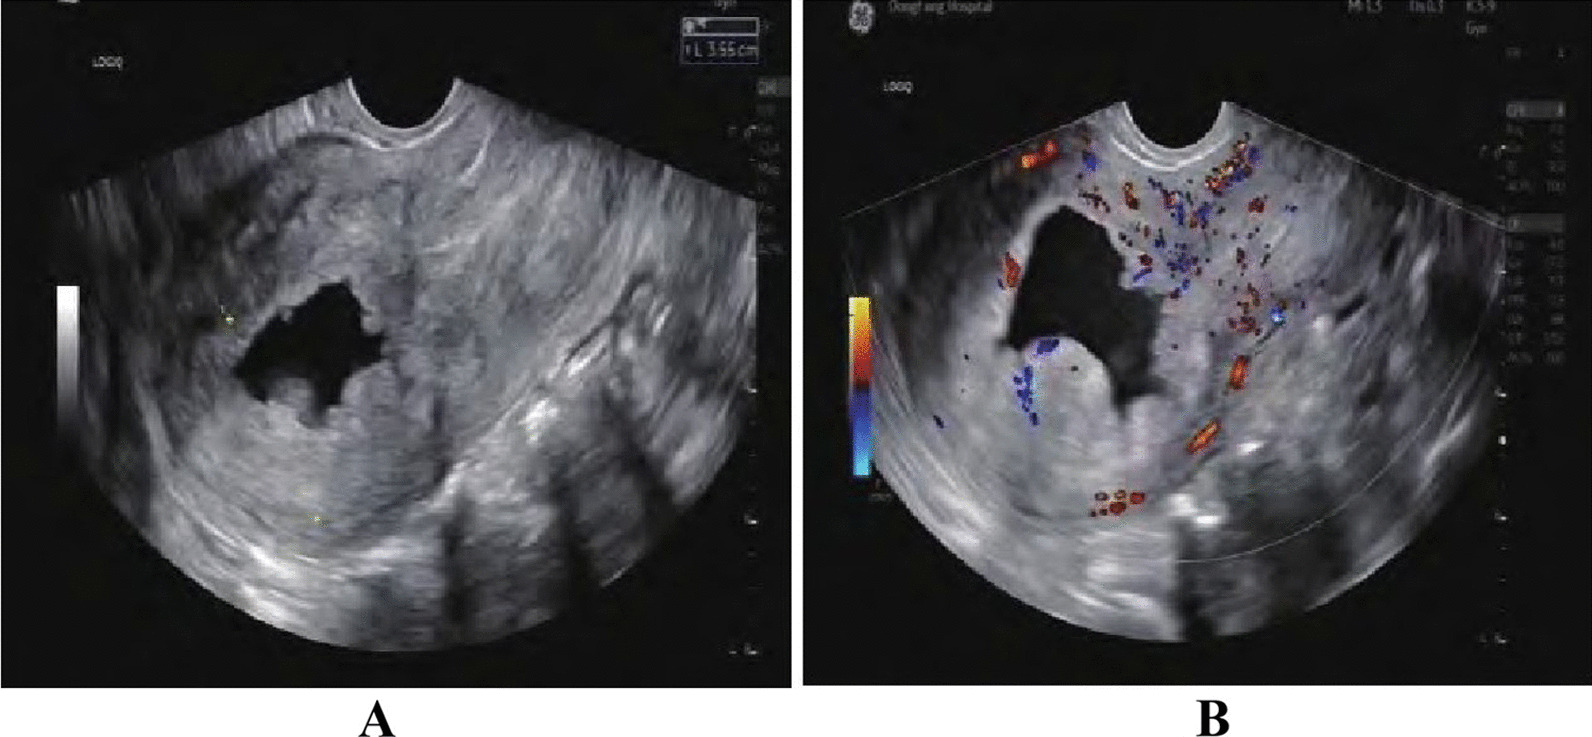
Imaging picture of a patient with endometrial cancer on transvaginal colour Doppler ultrasound. The patient was a female, 49 years old, with clinical presentation of irregular vaginal bleeding. The endometrial echogenicity was observed in 2D colour Doppler ultrasound, which showed that the endometrial echogenicity was heterogeneous and chaotic, with irregular endometrial morphology and contour, blurred echogenicity of the baseline, and unclear demarcation with the local myometrium, see A. Colour Doppler blood flow imaging showed rich blood flow signals, and the diagnosis of endometrial cancer was made, see B
